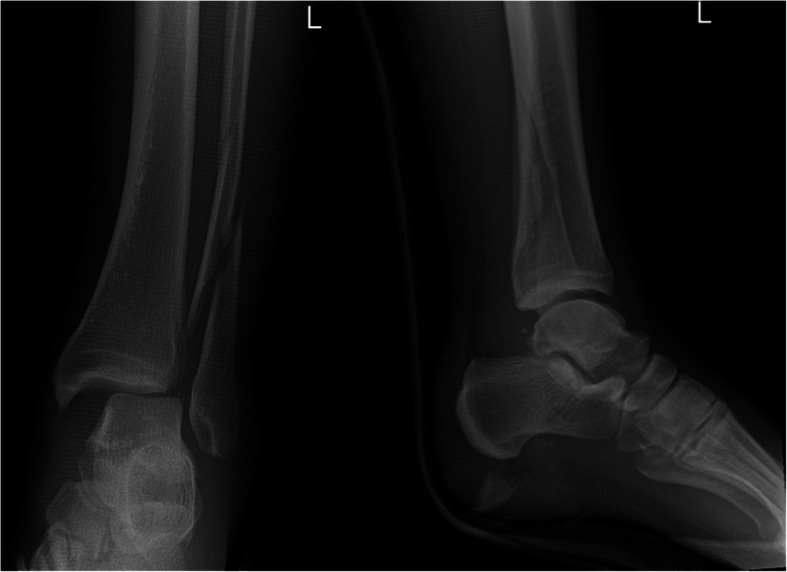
Anteroposterior and lateral plain ankle radiographs of the initial injury showing a Weber C ankle fracture subluxation with widened medial clear space, increased tibiofibular clear space, decreased tibiofibular overlap and lateral subluxation of the talus
